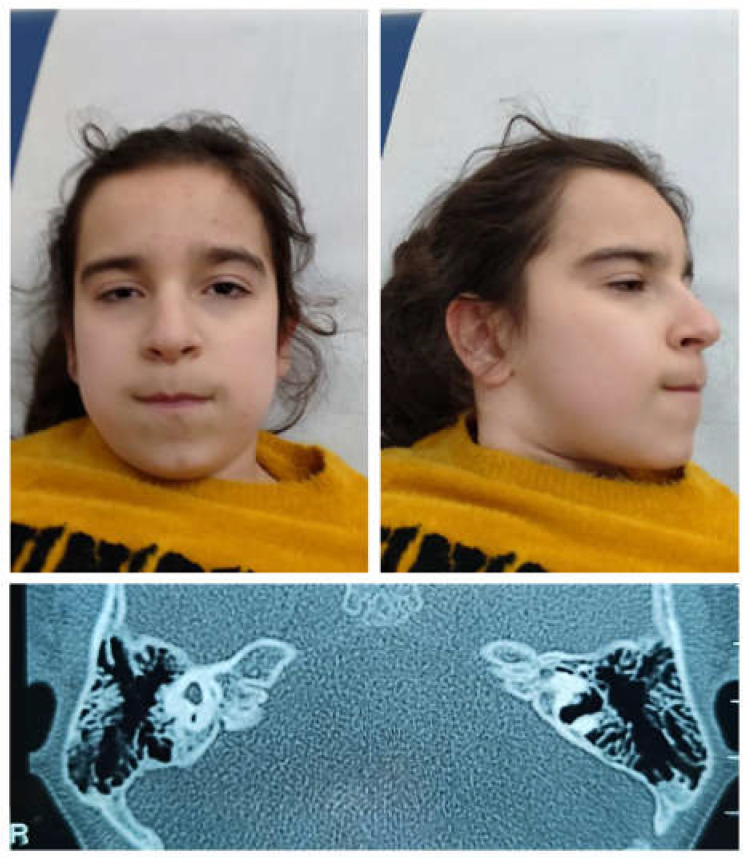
Clinical features of the subject carrying the missense *HNRNPC* pathogenic variant predicting the Arg99Gln amino acid substitution. Facial features (top panels). Note a broad and slightly receding forehead, high hairline, thick eyebrows, narrow and wave-shaped lids, bilateral microphthalmia, sharp nose, long philtrum, thin upper lip, prominent malar region, dimple chin, slightly posteriorly rotated ears, large triangular dimple, and fleshy lobes. CT scan of mastoids and petrous bone (bottom panel). Note aplasia of the left internal auditory canal (IAC), marked hypoplasia of the right IAC, and severe malformation of the vestibule, semicircular canals, and cochleae, bilaterally.
